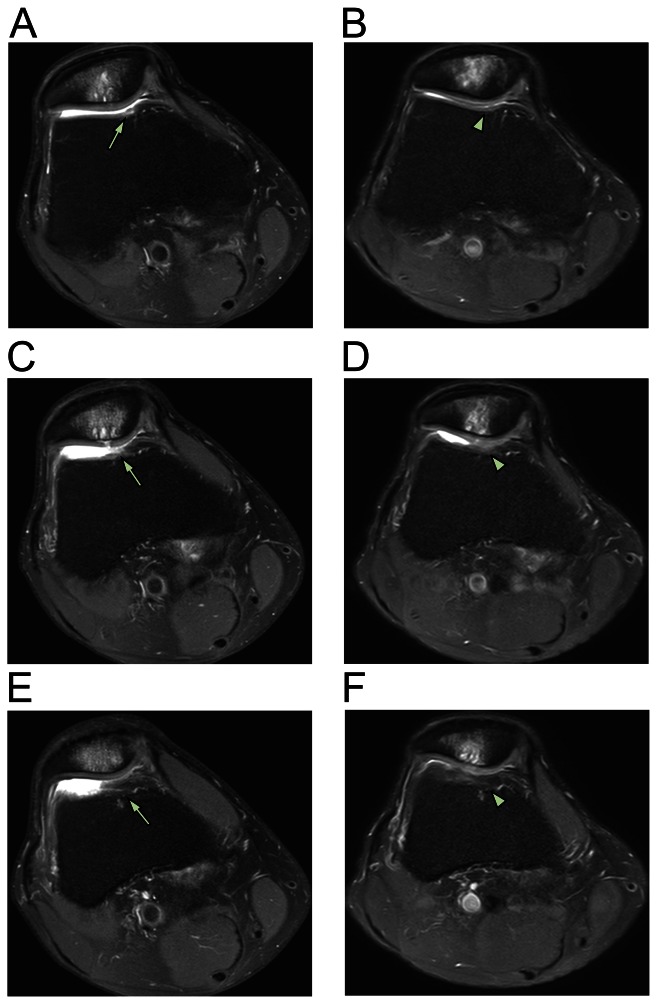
MRI axial sequential T2 views of patient 2. Pre-treatment MRI scans of patient 2 (A [16/24], C [17/24], E [18/24]) show retro-patellar signal changes (arrow) consistent with chondromalacia patellae (upper bone). At three months, post-treatment MRI scans of patient 2 (B [right; 4/20], D [5/20], and F [6/20]) show changes (arrowhead) consistent with probable cartilage restoration on the patellae-femoral joint.
